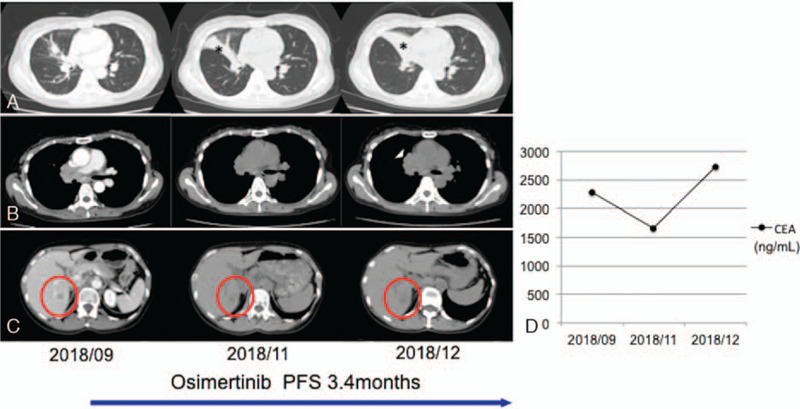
Clinical images and CEA monitoring during the treatment course. (A) CT images of the thorax showing tumor progression and atelectasis after 3.4 months of osimertinib treatment (asterisk). (B) CT images of the thorax showing multiple stable lymph node metastases (arrowhead). (C) CT images of the abdomen revealing a size increase in the liver metastasis (red circle). (D) CEA levels showing disease progression with osimertinib treatment. CEA = carcinoembryonic antigen; CT = computed tomography.
